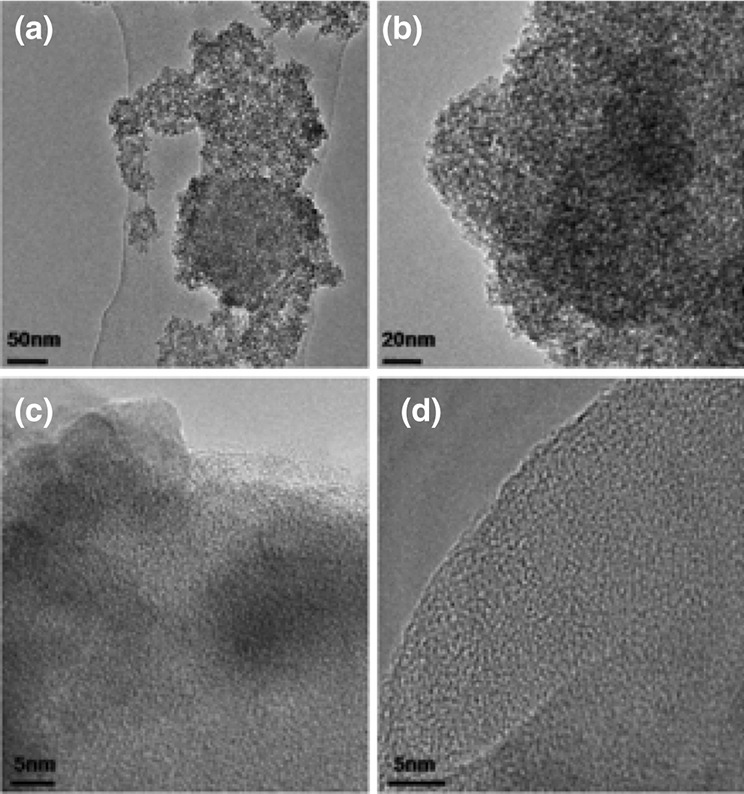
TEM and HRTEM images of non-ultrasound-treated white TiO_2_ (a, c) and amorphous hydroxylated black TiO_2_ obtained after 8 h of ultrasonication (b, d). Reprinted with permission from [89]. Copyright (2015) Springer Nature
